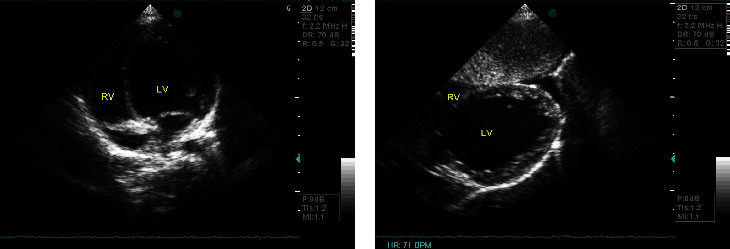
(a) Echocardiography from apical 4-chamber view: dilated left ventricle with global shape. (b) Subcostal view: noncompaction features at the apex. LV: left ventricle and RV: right ventricle.
